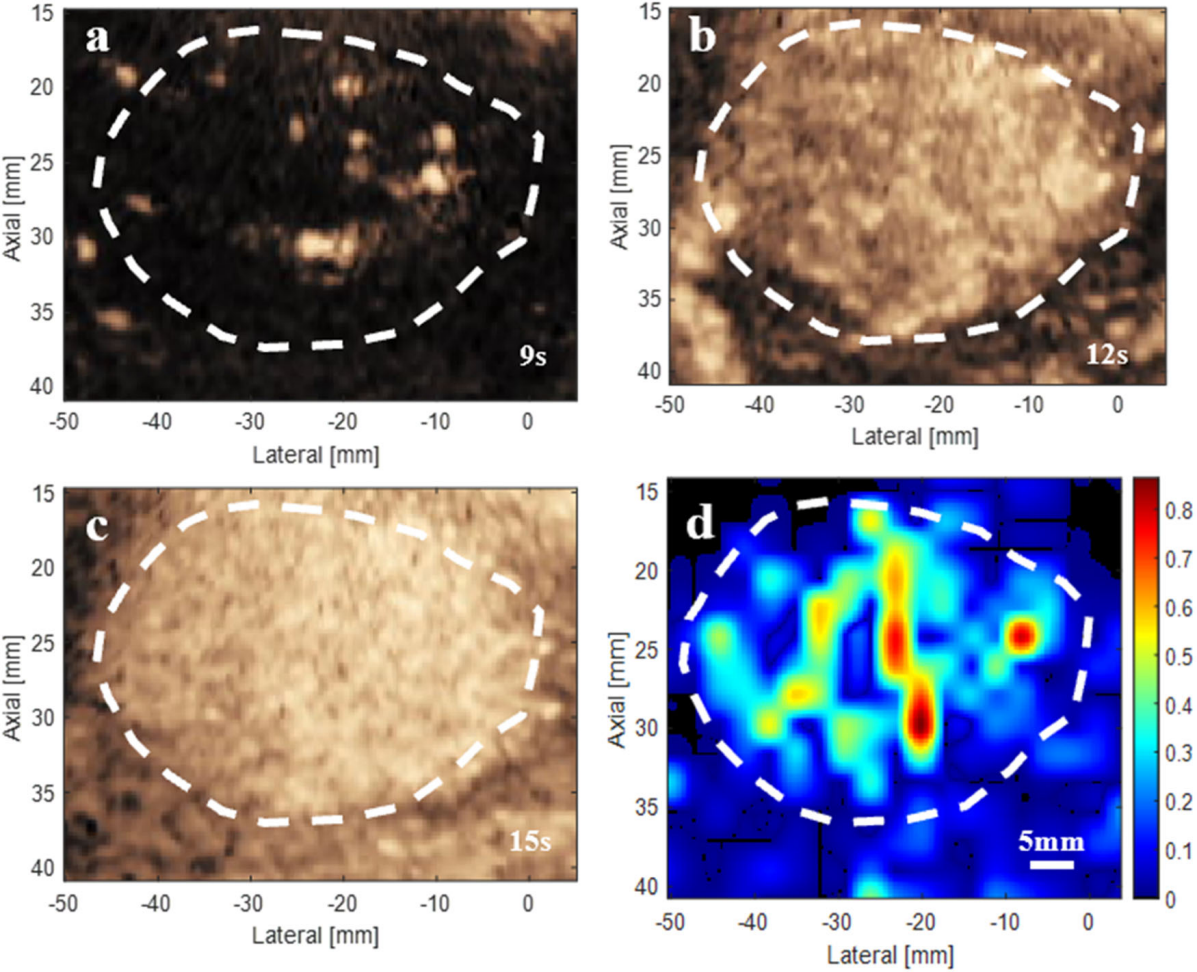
Contrast-enhanced ultrasound (CEUS) image and super-resolution ultrasound (SR-US) perfusion map of a 71-year-old female patient with hepatocellular carcinoma. a–c CEUS image. d The corresponding SR-US perfusion map. The white dashed line indicates the lesion boundary
